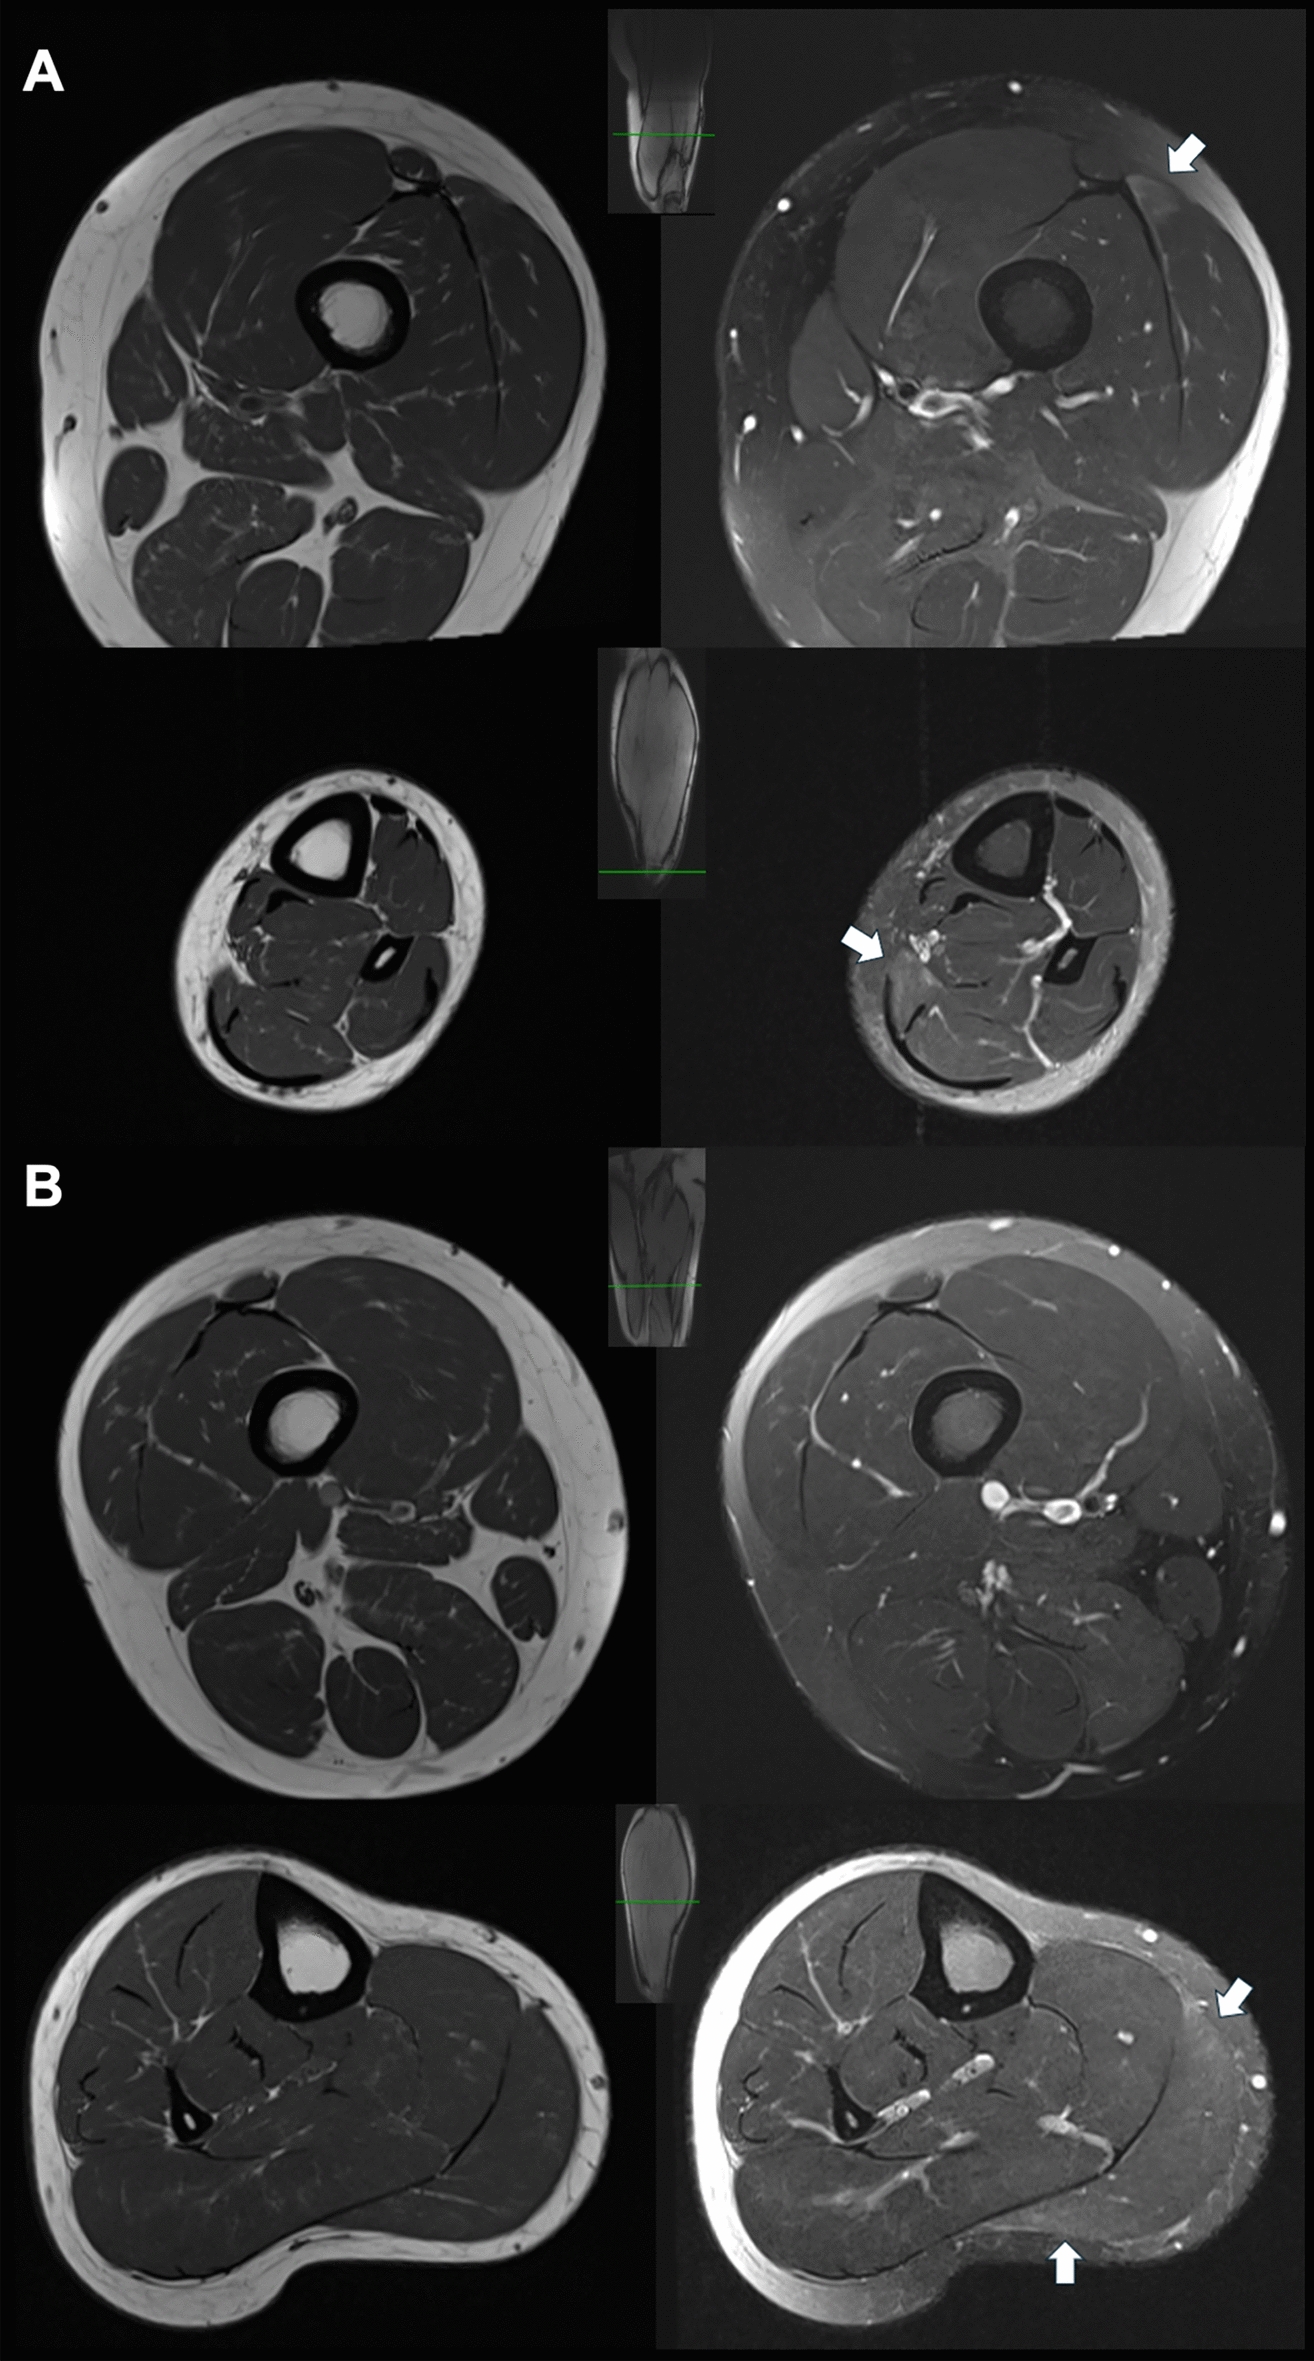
Patient 2's MRI images. A Left leg. Left images are T1 non-contrast axial MRI of the lower thigh (top) and lower leg (bottom) revealing no fatty infiltration. Right sided images are T2 STIR MRI of the right lower thigh (top) revealing subtle T2 hyperintensity in the vastus lateralis (arrow) and lower leg (bottom) revealing T2 hyperintensity in the soleus (arrow). B Right leg. Left images are T1 non-contrast axial MRI of the lower thigh (top) and of the lower leg (bottom) showing no significant fatty infiltration. Right sided images show non-contrast axial T2 STIR MRI of the right lower thigh (top) showing no significant abnormalities and lower leg (bottom) revealing T2 hyperintensity in the medial gastrocnemius (arrow)
